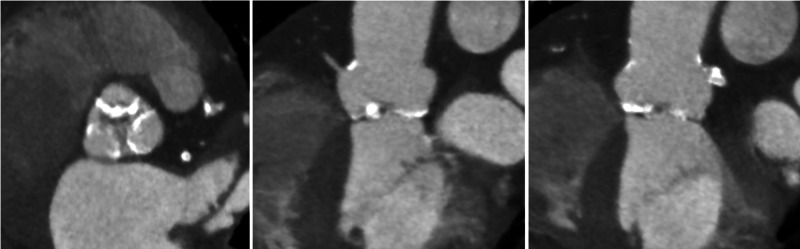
Multislice computed tomographic scan showing heavily calcified trileaflet aortic valve.
